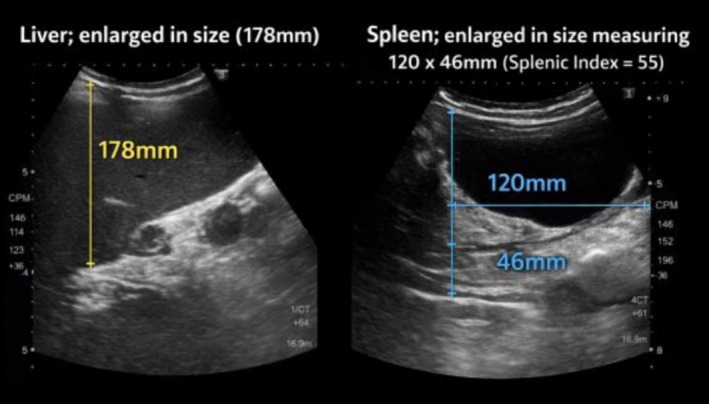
Abdominal ultrasound demonstrating hepatosplenomegaly, with enlarged liver and spleen consistent with dengue‐associated visceral involvement.
